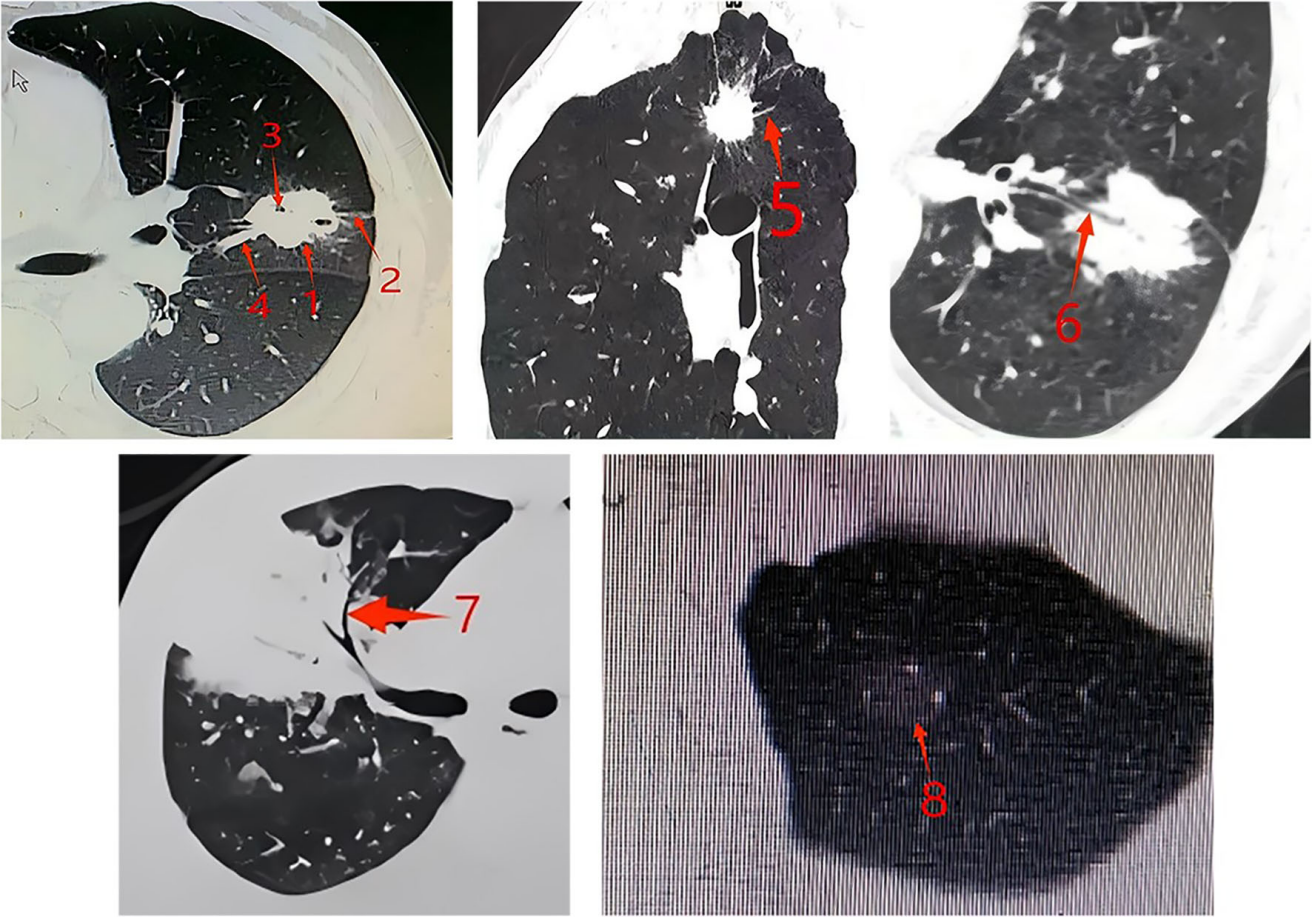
CT features of tumors. Arrow 1: Lobulation sign: The surface of the nodule is uneven, with multiple arc-shaped depressions visible on it, resembling the combined appearance of multiple nodules. Arrow 2: Pleural indentation sign: The distal pleura of the tumor is pulled by the tumor, forming a linear high-density shadow. The corresponding concave area shows a trumpet-shaped change. Arrow 3: Vacuole sign: Round, transparent shadows with a diameter of less than 5mm (mostly 1-2mm) can be seen within the lesion, either singly or in multiple clusters, and they can be located anywhere within the lesion. Arrow 4: Vessel convergence sign: Around the tumor, there is one or more blood vessels that converge towards the tumor. It can also be manifested as the blood vessels stopping or being completely surrounded and destroyed when they reach the edge of the tumor. Arrow 5: Spicule sign: The tumor margin extends outward, the base is slightly thick and gradually becomes thinner as it spreads outward, presenting a straight and forceful linear shadow. Arrow 6: Bronchial cutoff sign: The bronchus is interrupted at the edge of the lesion or as it enters the lesion. The terminal end of the lumen may be straight, blunt, or conical narrowed. The bronchial wall is locally thickened and asymmetric, gradually thinning towards the center of the lesion. Arrow 7: Air bronchogram: There are long, branched or tubular low-density shadows within the lesion, which may be accompanied by blood vessels. When perpendicular to the scanning plane, they can appear as continuous circular low-density shadows on the upper and lower layers. Arrow 8: Ground-glass nodules: The tumor nodules have a relatively low density in part or the entire area, presenting as ground-glass opacity, without obscuring the pulmonary vascular patterns, and the lesion boundaries are clear.
